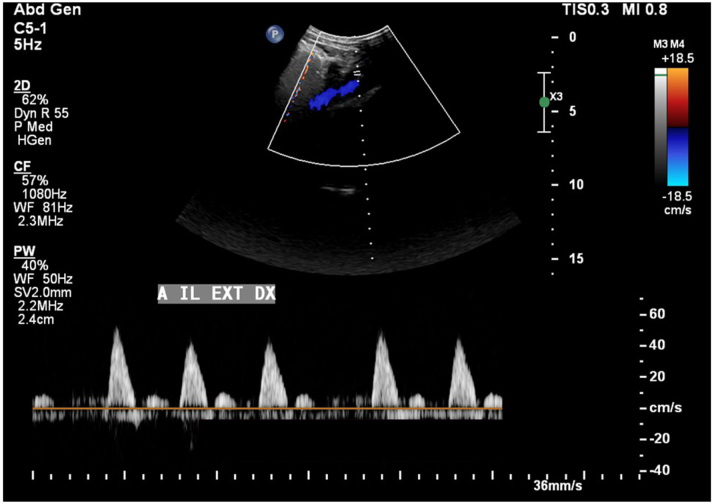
2-Year Follow-Up Post Percutaneous Transluminal Angioplasty Distal Aortic Intervention Through Duplex Bilateral Common Iliac Artery Ultrasound
